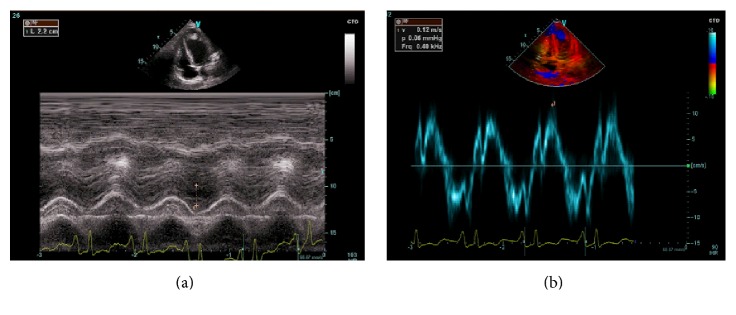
Transthoracic echocardiography, tissular Doppler showing a preserved function of the right ventricule: (a) TAPSE at 22 mm, (b) tricuspid lateral annular systolic velocity wave (S′) at 0.12 m/s.
